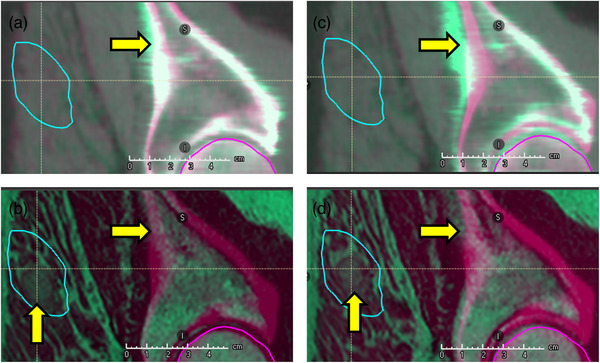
Example of CTgRT misalignment from Patient 4. When aligning the daily synthetic CT to the reference CT (a), the lesion is not visible, but the bony structures and external contour can be aligned. When applying this registration transformation to the daily clinical MRI (b), it is apparent that the lesion is not centered within the PTV and resides outside of the higher dose GTV (contour not shown for lesion visibility). When the daily clinical registration transformation is applied to the daily synthetic CT (c), the bony structures and external contour are misaligned, and the lesion is still not visible. When applying this registration transformation to the daily clinical MRI (d), the lesion is centered appropriately within the PTV (and GTV) contours.
